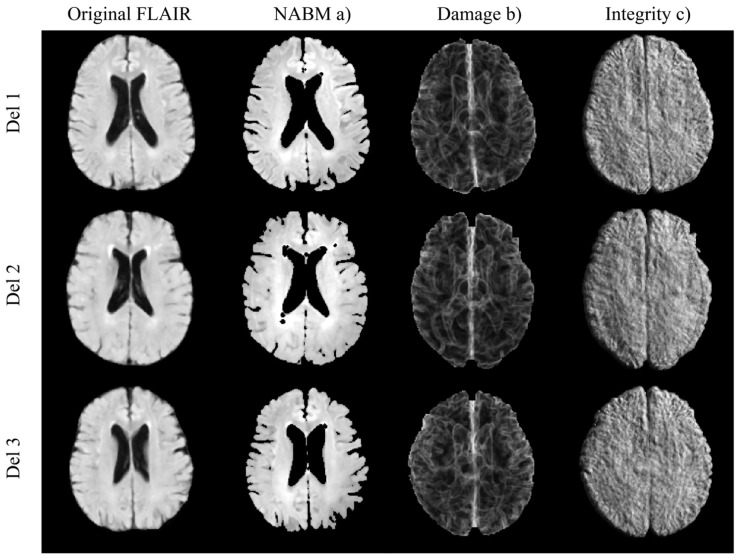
FLAIR MRI slices for original, NABM (a) and Damage (b) and Integrity markers (c).
